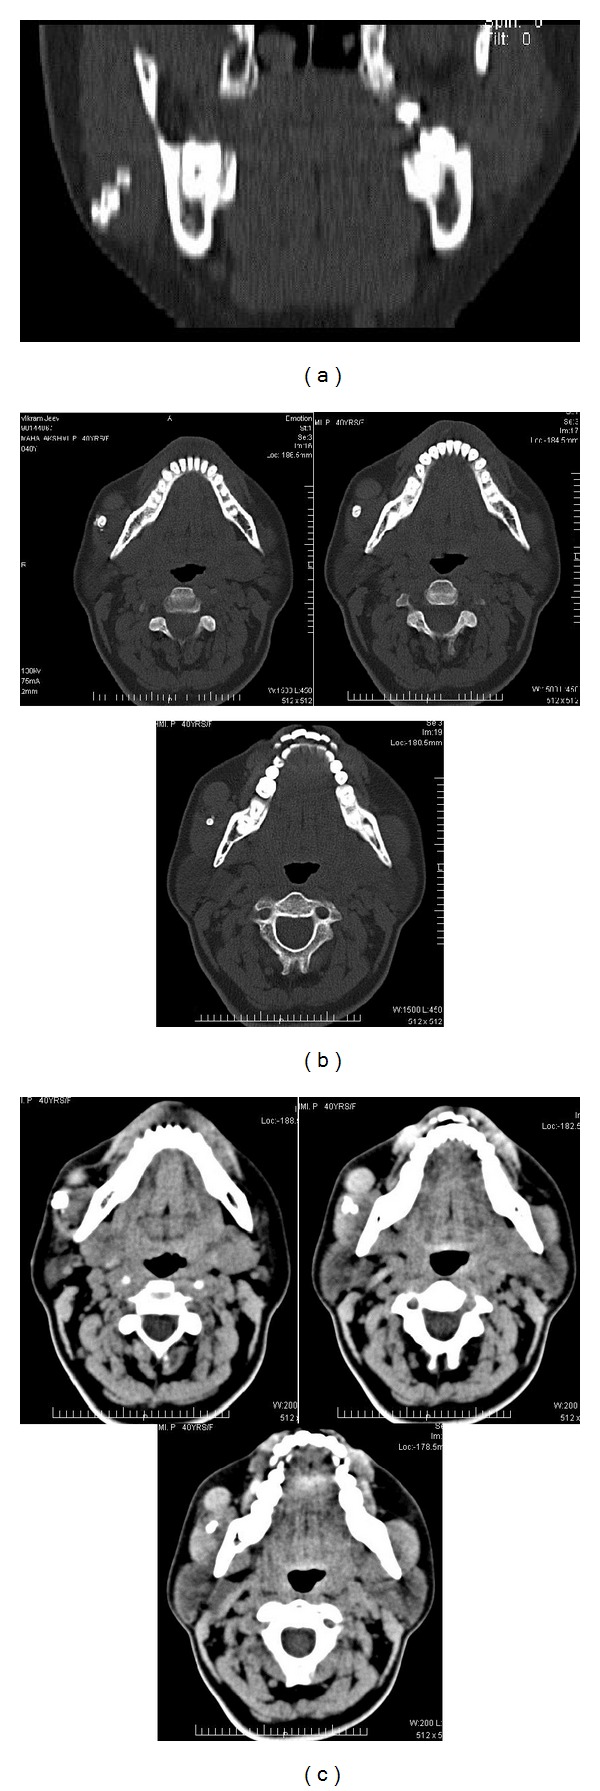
(a) Positions of the calcific structures noted in coronal CT section. (b) Bone window axial CT image shows position of the calcific structures at various depths. (c) Soft tissue window axial CT image clearly showing the boundaries of the mass.
